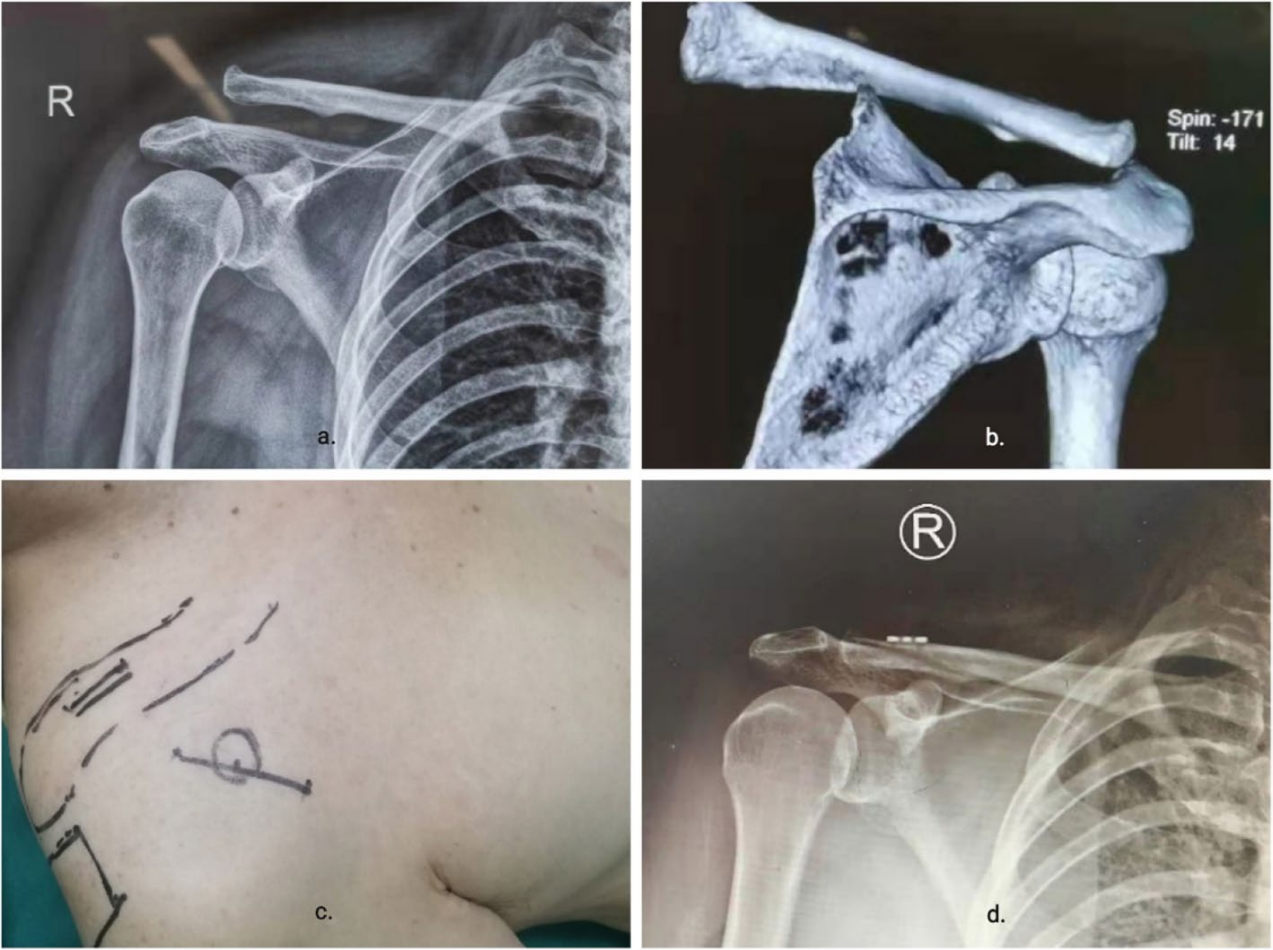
Introduction to the perioperative period. a, b Pre-operative X-ray and CT evaluation and measurement of acromioclavicular joint dislocation. c Primary body surface location and marking by imaging and anatomical position. d Postoperative imaging assessment of reduction
